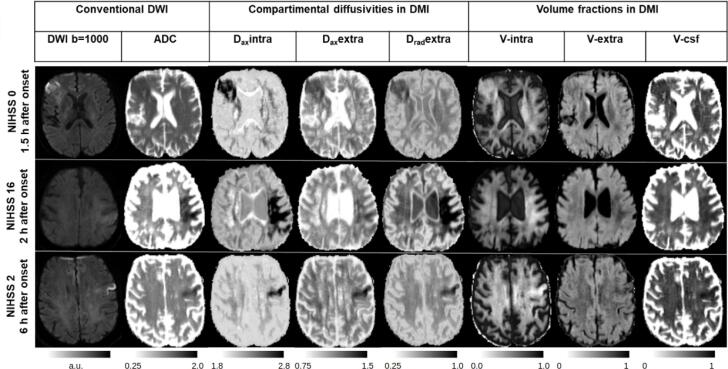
Conventional DWI (left) and DMI maps (right) shown in 3 exemplary cases. Similar qualitative DMI changes were observed within infarct lesions. Diffusivities were decreased in all compartments, with D_ax_intra showing the strongest effects. Regarding the volume fractions, the increase in V-intra is contrary to the decrease in V-extra and V-CSF, indicating net swelling within the intra-axonal compartment. Multi-parametric DMI maps appear to provide a more differentiated, pronounced picture of tissue alterations in stroke compared to that elicited by conventional DWI.
